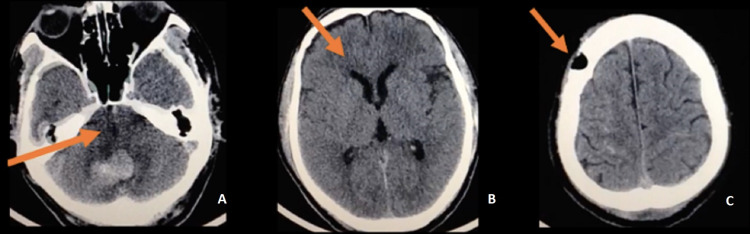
Postoperative non-contrast CT scan (A) Axial view orange arrow shows a central and open fourth ventricle. (B) Orange arrow shows the resolution of hydrocephalus. (C) Orange arrow shows the minimally invasive approach through Kocher's point.
